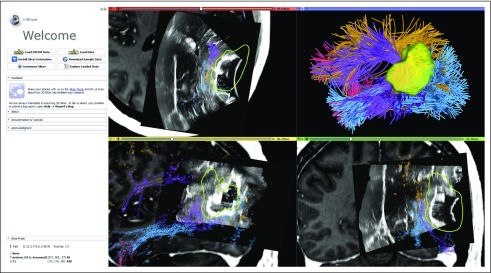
The 3D Slicer user interface that shows visualization of navigated intraoperative ultrasound (iUS) overlaid on preoperative T1-weighted magnetic resonance imaging with contrast along with the automatically identified language fiber tracts in patient data set 2 (tracts and colors as in Fig 4). The iUS image is used retrospectively here for a demonstration of software functionality. iUS information demonstrates brain shift that occurs after dural opening and likely displaces the arcuate fasciculus (purple), which can be seen at the edge of various parts of the resection cavity in each panel (top left, axial; bottom left, sagittal; bottom right, coronal). By integrating this brain shift information with tractography, the surgeon can make an updated estimate of how far the tracts might be. DICOM, Digital Imaging and Communications in Medicine.
